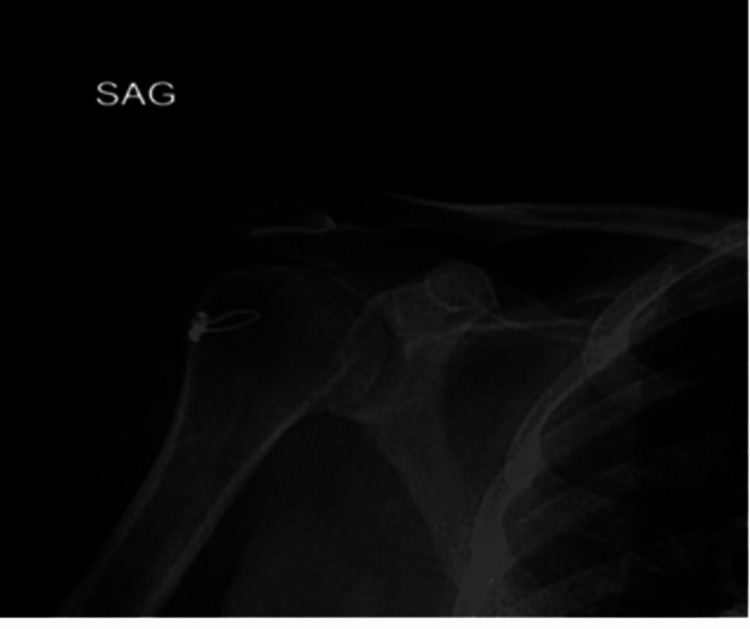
Postoperative antero-posterior shoulder radiograph
